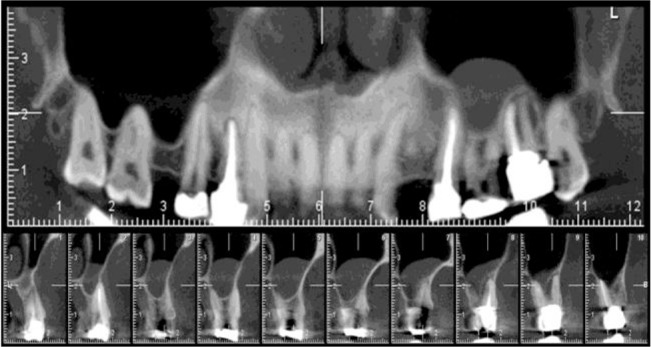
Mucous retention pseudocyst (MRP) of the left maxillary sinus on cross-sectional and panoramic-like CBCT views
